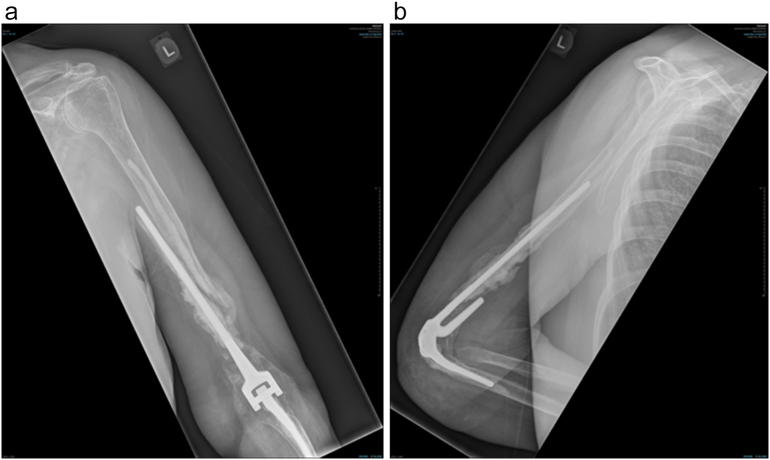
Pre-operative X-ray in 2024, after the previously revised TEA. Anteroposterior [A] and lateral [B] views show the re-loosening of the humeral prosthesis together with significant bone loss and complete perforation of the cortical bone. No ulnar loosening is present.
